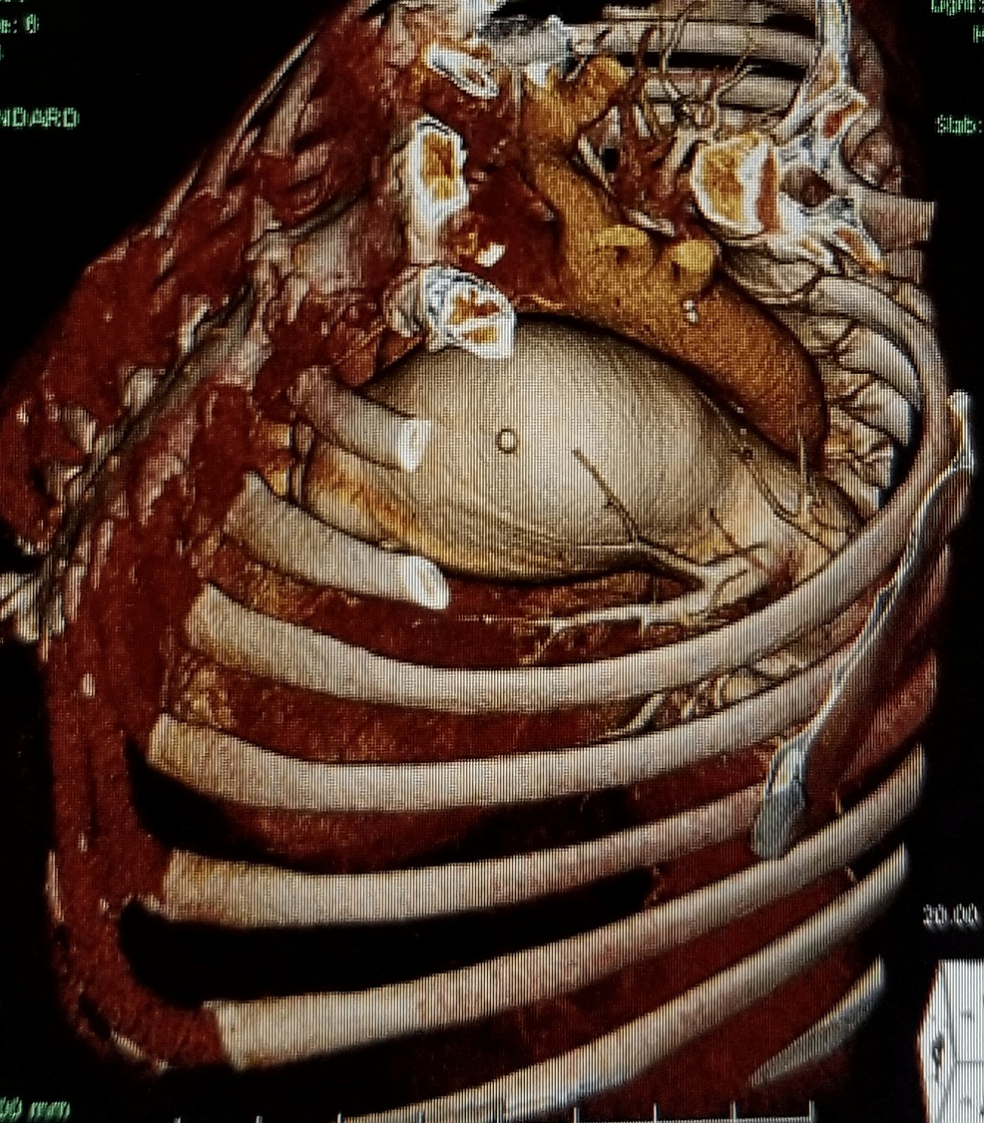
Severe pulmonary artery aneurysm as visualized on CTA with 3D reconstruction
